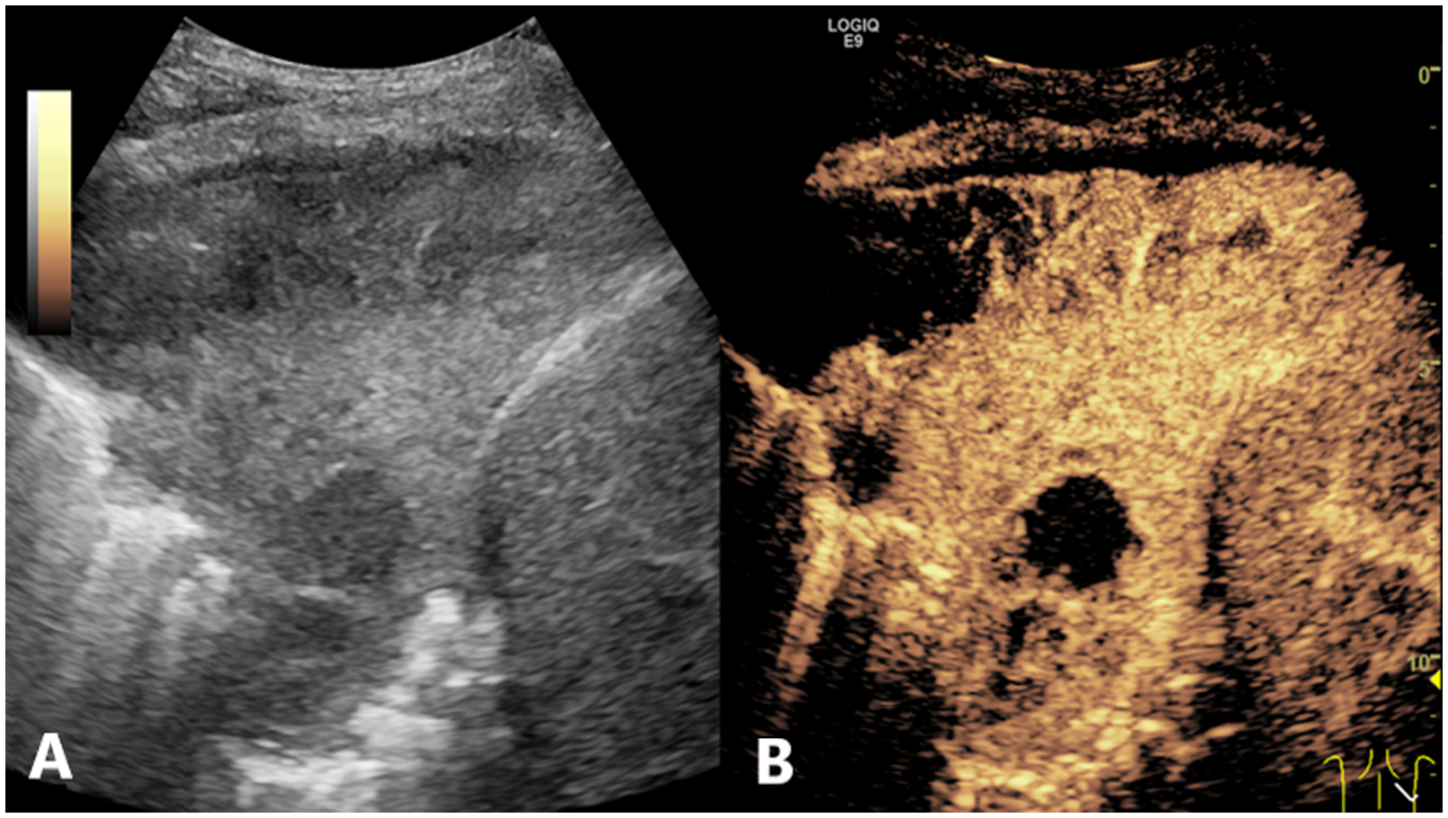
Conventional US and CEUS images of a subpleural pulmonary lesion (LUSC) in the lower lobe of right lung of a 62-year-old male. Conventional US (A) showed an isoechoic lesion with a maximum diameter of 9.8 cm. The lesion exhibited an irregular shape and a heterogeneous composition. CEUS (B) demonstrated a heterogeneous pattern of hyper-enhancement in the lesions, accompanied by the presence of perfusion defects. The serum CEA result was negative. The probability of LUSC, as calculated by the prediction model, was approximately 0.9, which was greater than the cutoff value (0.375). This indicated that the lesion was predicted to be LUSC, which was consistent with the definitive diagnosis. US, ultrasound; CEUS, contrast-enhanced ultrasound; LUSC, lung squamous cell carcinoma; CEA, carcinoembryonic antigen.
